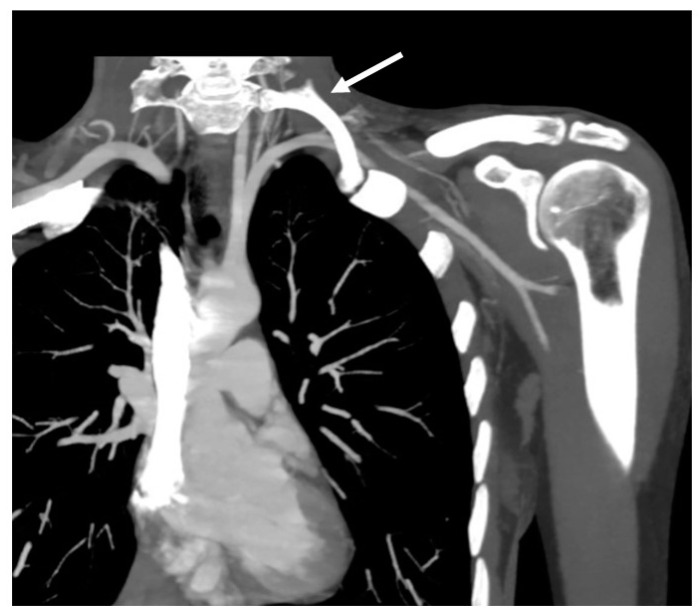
CT scan disclosing left subclavian artery compression by complete supernumerary cervical rib (white arrow).
